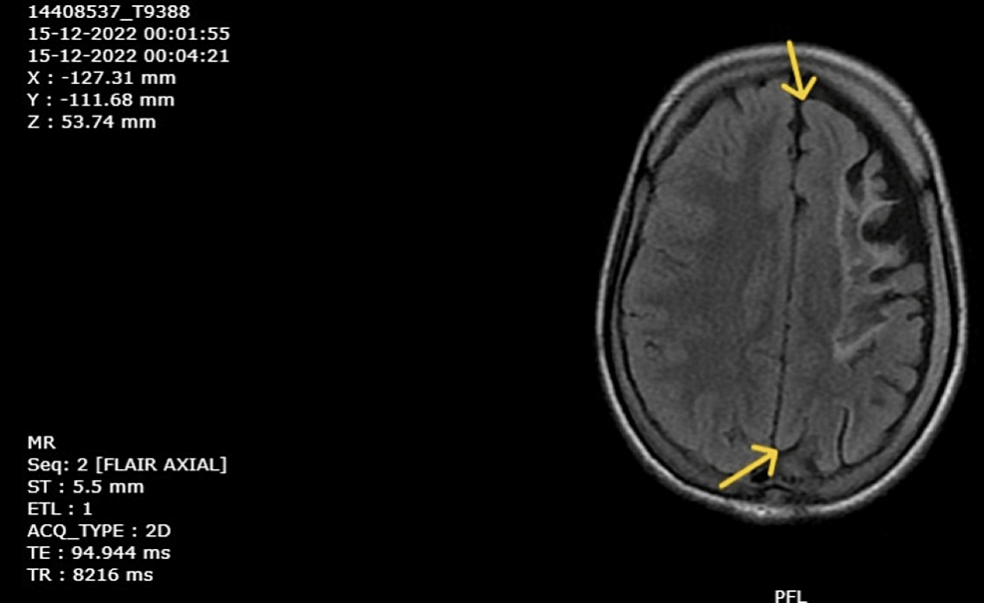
MRI of brain showing, paradoxical midline shift towards left side (December 10, 2022).
